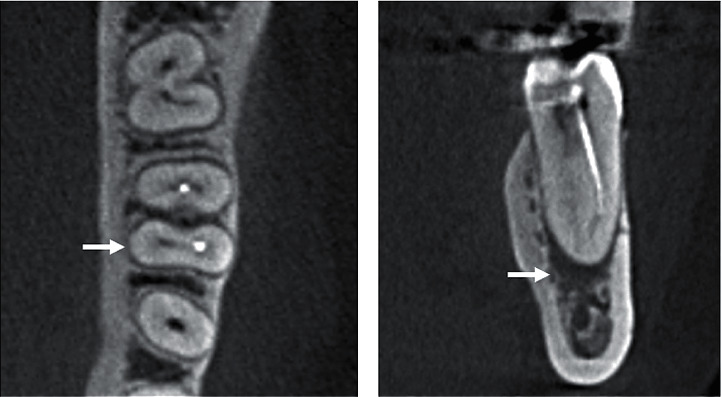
CBCT slices of the mandibular first molar: (a) axial section with the missed ML canal (arrow); (b) coronal section with the missed ML canal and the presence of apical radiolucency (arrow).
